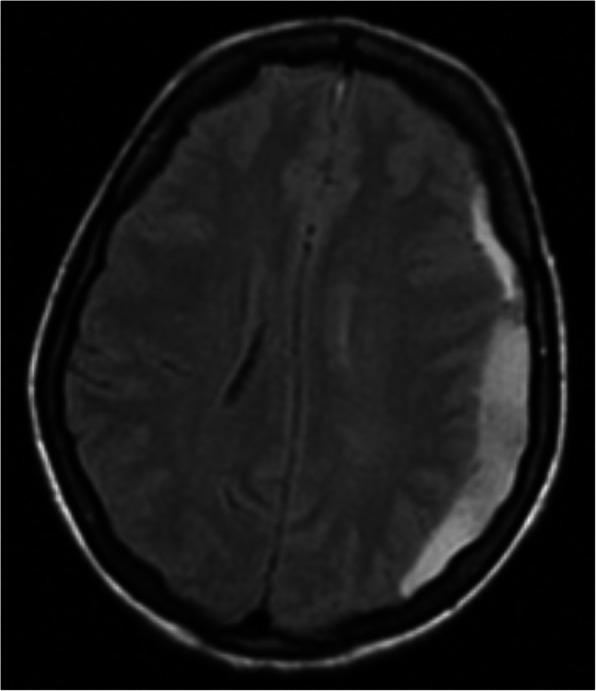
Axial T2-weighted-Fluid-Attenuated Inversion Recovery (FLAIR) image shows left subdural hematoma causing slight right midline shift
